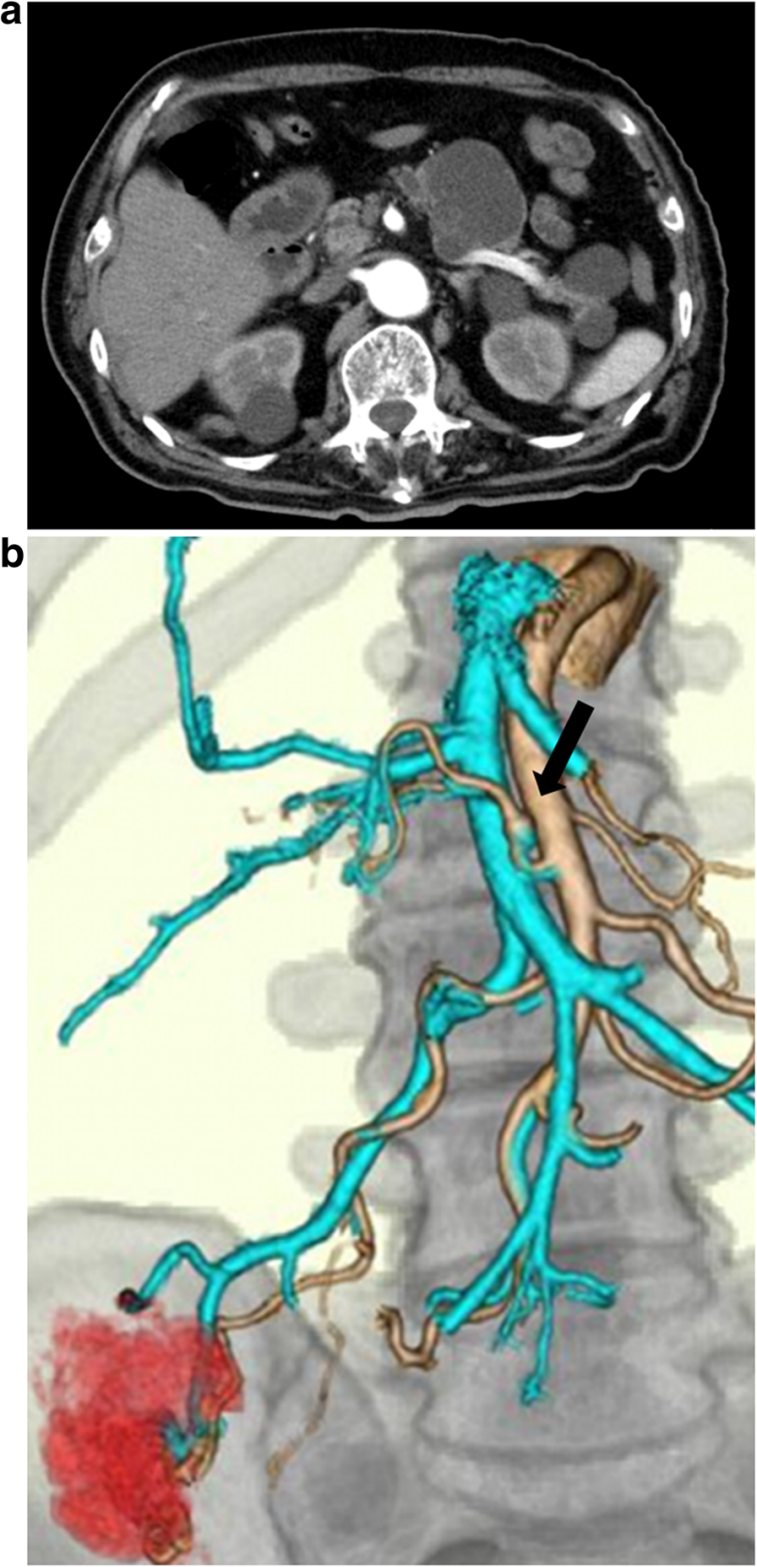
Preoperative computed tomography (CT) scan imaging (a) and CT angiography (b) showing a middle colic artery aneurysm (black arrow) measuring 10 × 7 mm without extravasation.
